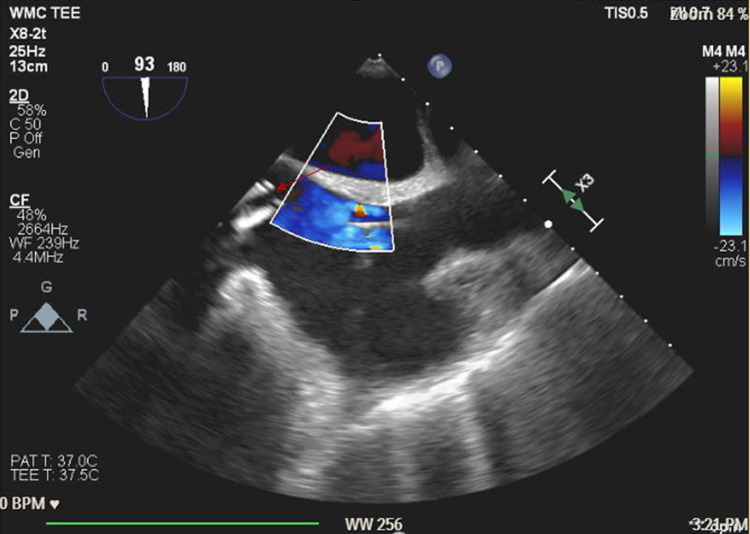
Perioperative TEE (mid-esophageal bicaval view) immediately after VA-ECMO femoral cannulation. Red arrow shows ECMO canula in the atriocaval junction. TEE: transesophageal echo; VA: veno-arterial; ECMO: extracorporeal membrane oxygenation
